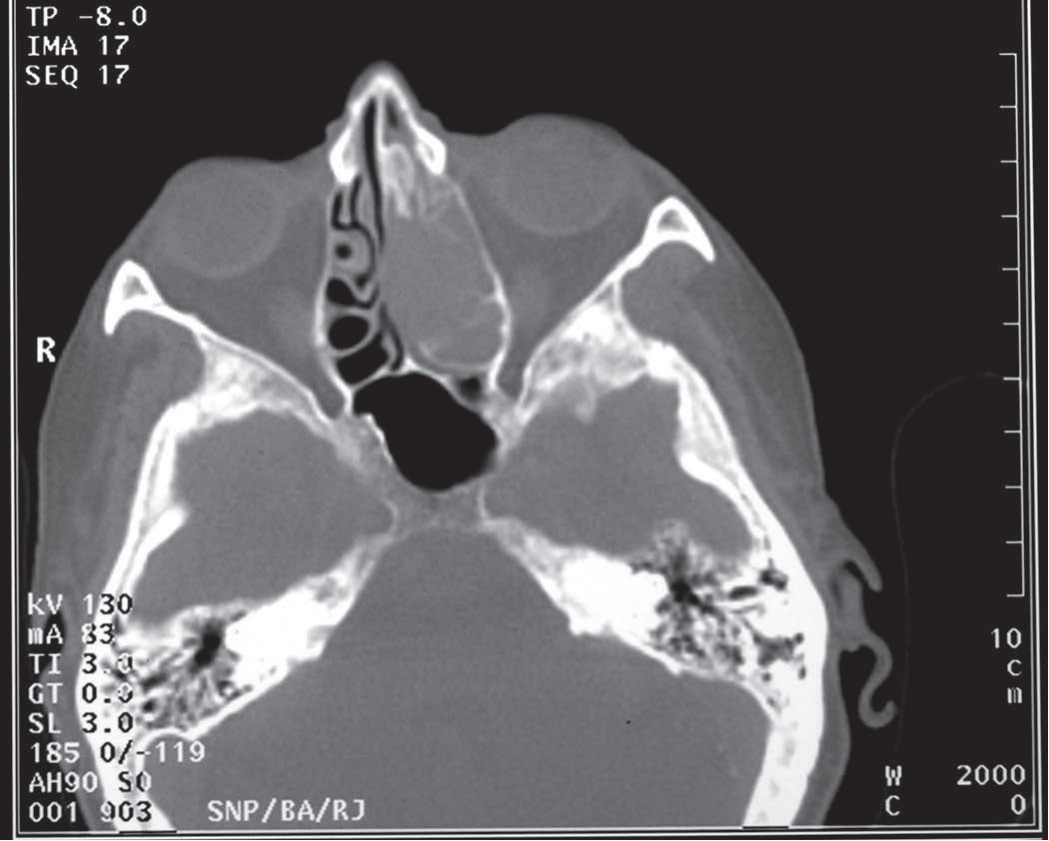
Axial CT scan of the nose and paranasal sinuses showing that the lesion occupies the left nasal cavity and ethmoid sinuses.
